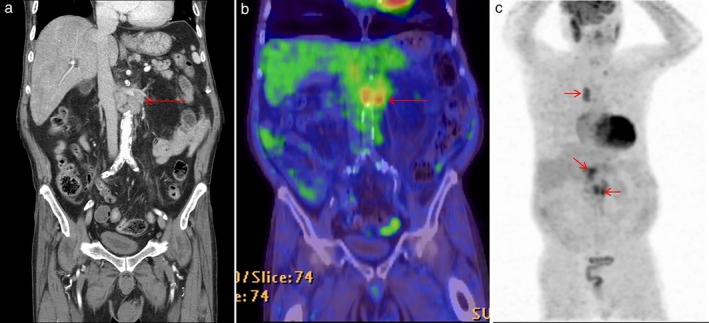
Positron emission tomography‐computed tomography (PET‐CT) scans. (a, b) Enlarged para‐aortic lymph nodes revealed hypermetabolic activity on PET‐CT. (c) Maximum intensity projection (MIP) image in PET‐CT showed no definite hypermetabolic mass in the chest and abdomen, except mediastinal and abdominal lymph nodes (arrows).
